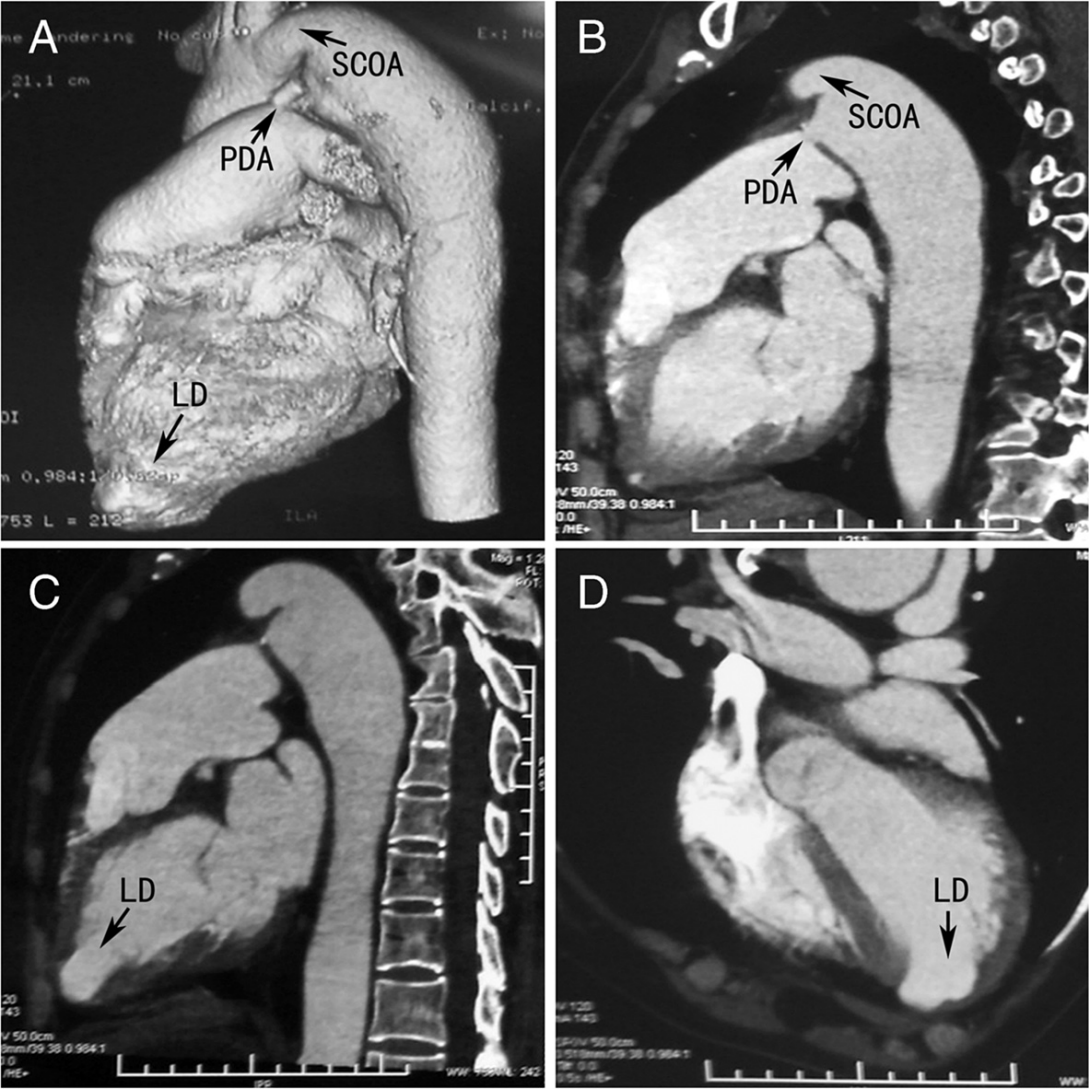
Helical CT showing a PDA, a LD at the cardiac apex and a SCOA of no collateral circulation (a to d)
